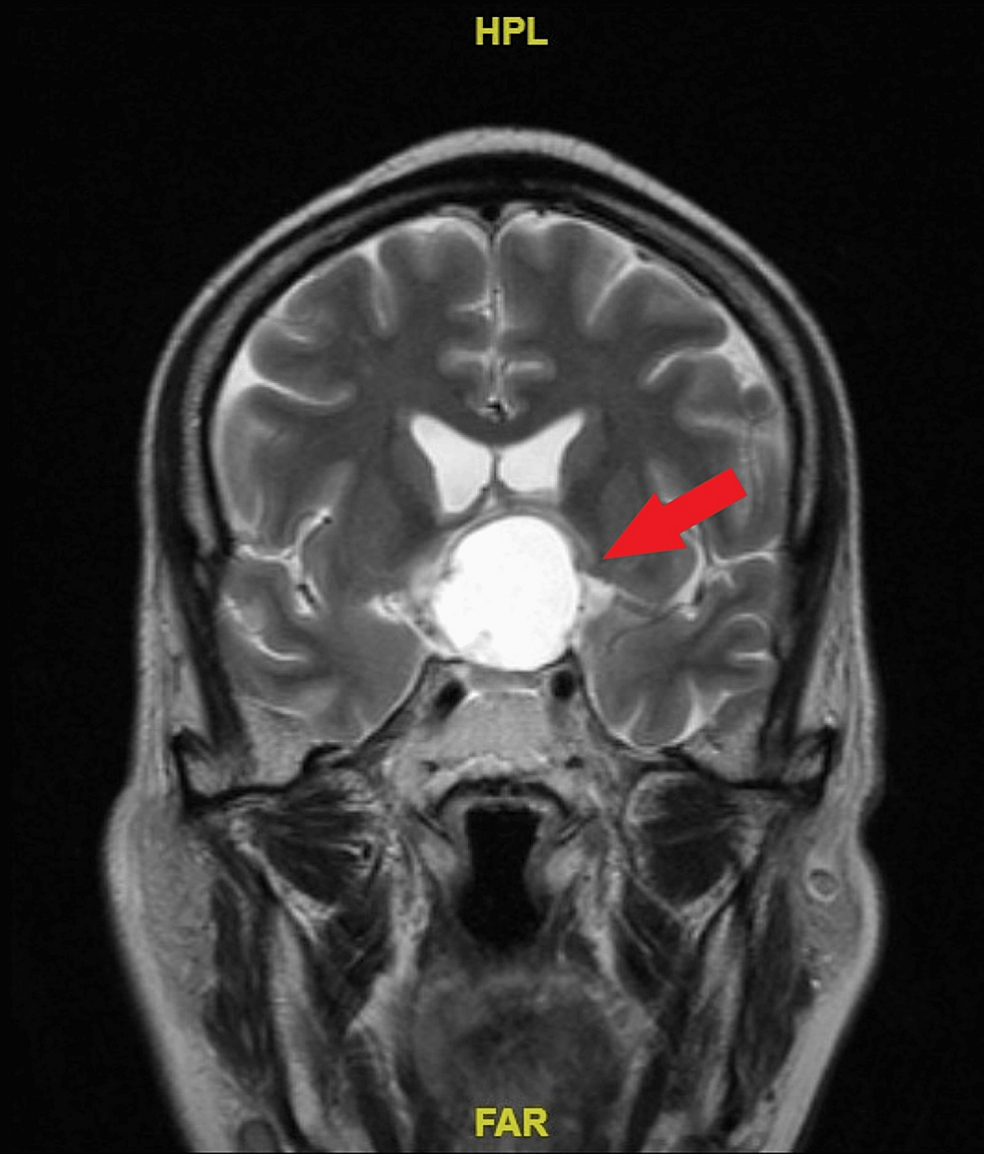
Coronal cut of the T2-weighted magnetic resonance imaging showed a well-defined hyperintense mass at the suprasellar area compressing the adjacent brain structure (red arrow).
